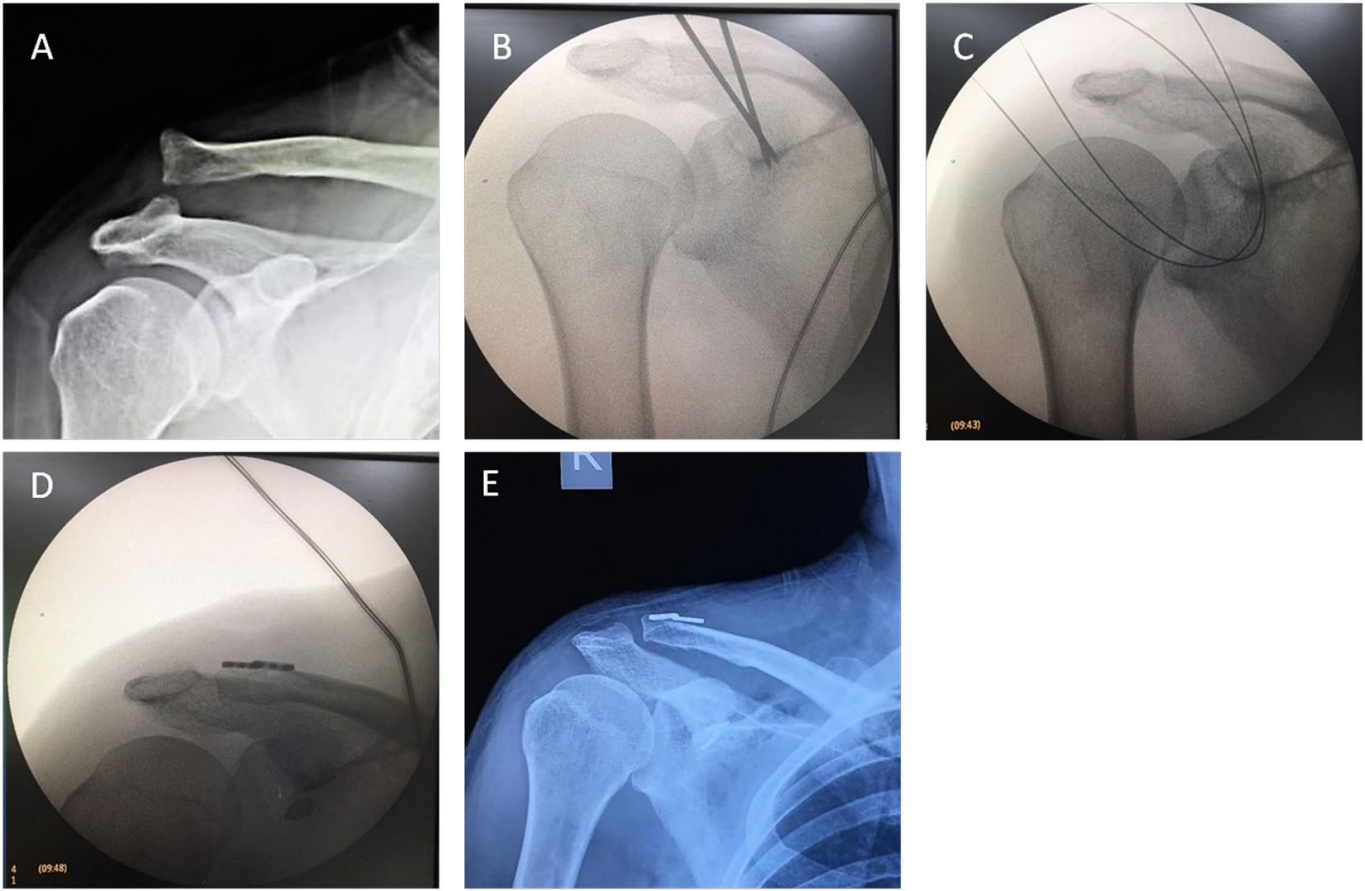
Pre- and post-operative radiographs of a right AC joint dislocation. (A) Preoperative X-ray of the right shoulder of a 46-year-old male patient who sustained a Neer type III AC joint dislocation after a fall. His coracoclavicular distance measured 29 mm, and his Constant score was 42. (B) Operative X-ray showing the 2 guide pins crossing from the clavicle to the coracoid base in place of the conoid and trapezoid ligaments. (C) Operative X-ray showing the 2 suture-passing wires cross through the clavicular and coracoid tunnels in place of the conoid and trapezoid ligaments. (D) Operative X-ray showing full reduction in the AC joint with the 2 TightRope systems reconstructing the trapezoid and conoid ligaments. (E) Post-operative X-ray of the right, dominant shoulder at the final follow-up 18 months after surgery demonstrating anatomical reduction of the AC joint with a coracoclavicular distance of 8 mm in both shoulders. His Constant score was 100.
